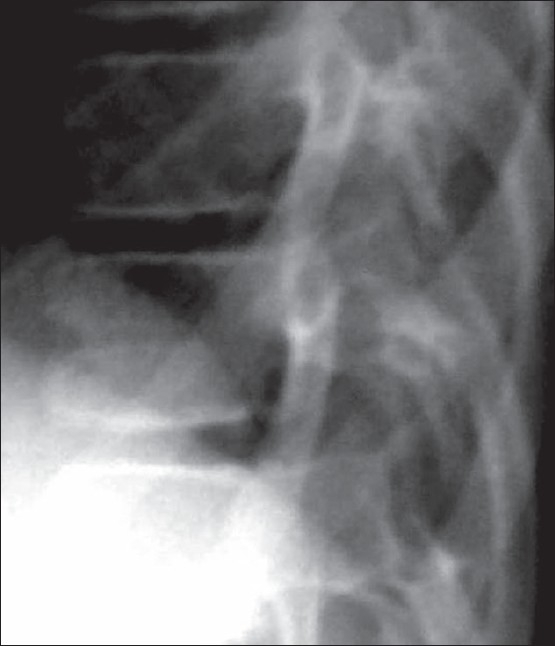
Focused lateral radiograph of the dorsal spine shows fishmouth vertebra
